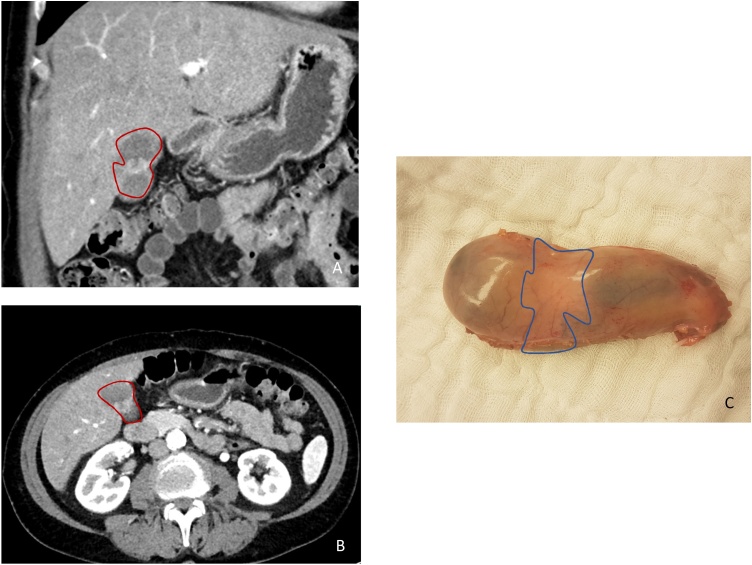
CT abdomen (portal venous phase) transversal plane (A), coronal plane (B) and macroscopic finding (C) with gallbladder (red) and metastasis (blue).
